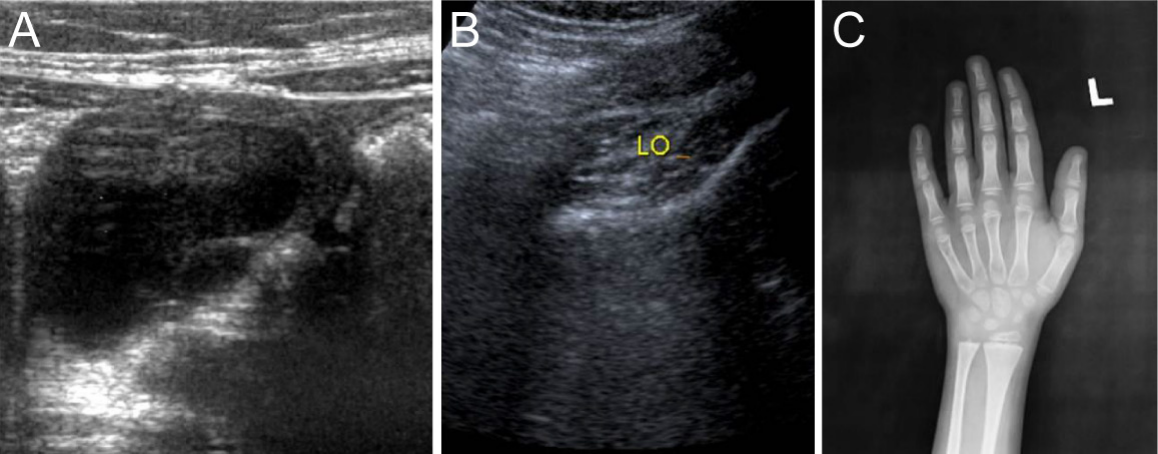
(A) Ultrasound abdomen at presentation with polycystic ovaries. (B) Ultrasound abdomen at follow-up with resolved cyst; she conceived and had two children. (C) P3 presented at 7 years with premature menarche but delayed bone age less than 6 years.
